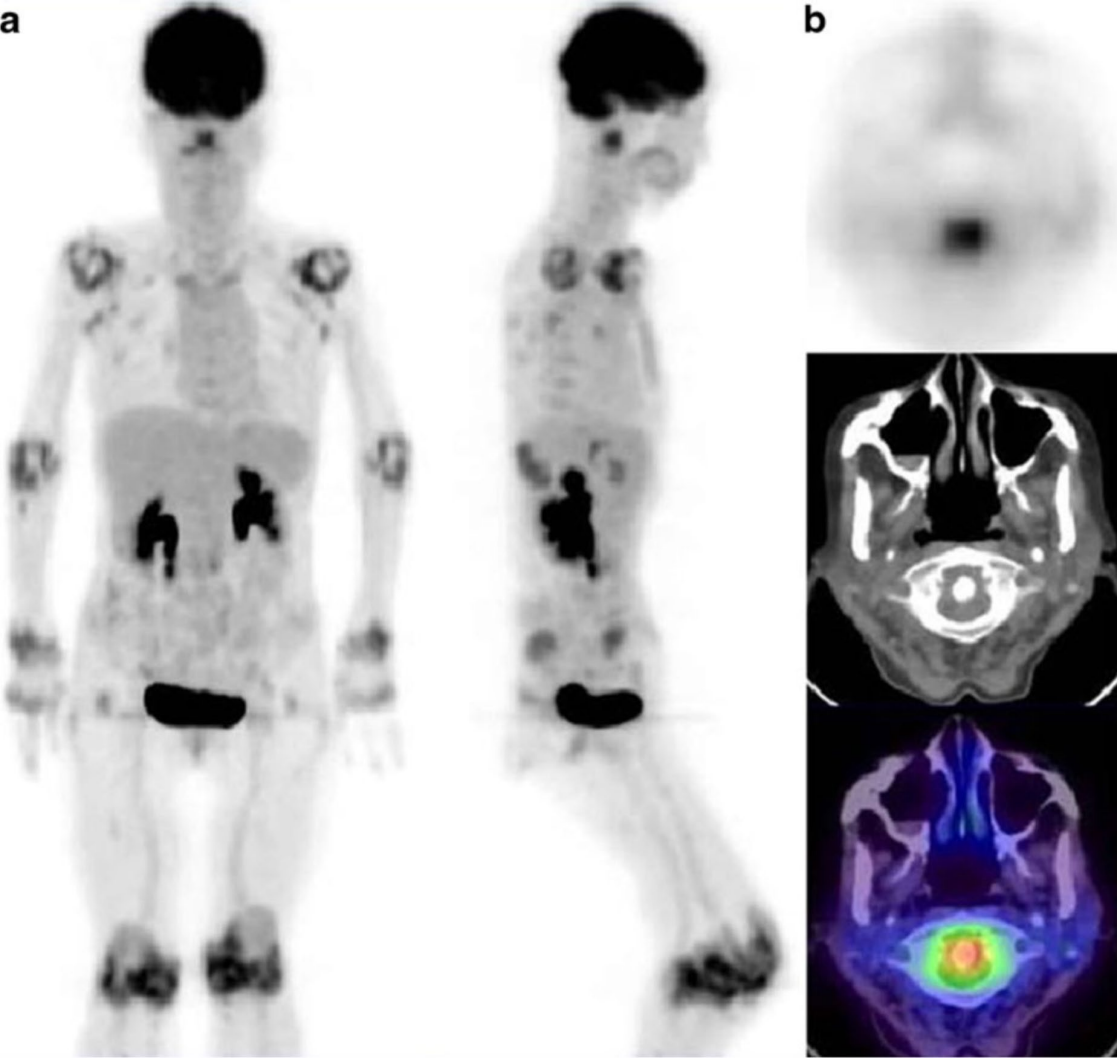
[^18^F]-FDG PET/CT was performed on a 71-year-old female with rheumatoid arthritis for seven years and was experiencing a flare-up. Maximum intensity projection (MIP) images of [^18^F]-FDG PET/CT in the anterior and right lateral views are shown in (a). Axial images of the atlantoaxial joint are shown in (b) (from top to bottom: PET, CT, and fused PET/CT). Atlantoaxial joint, right and left axillary lymph nodes, knees, hips, carpals, wrists, elbows, and shoulders all showed significant [^18^F]-FDG uptake. Reproduced with permission from [25]
